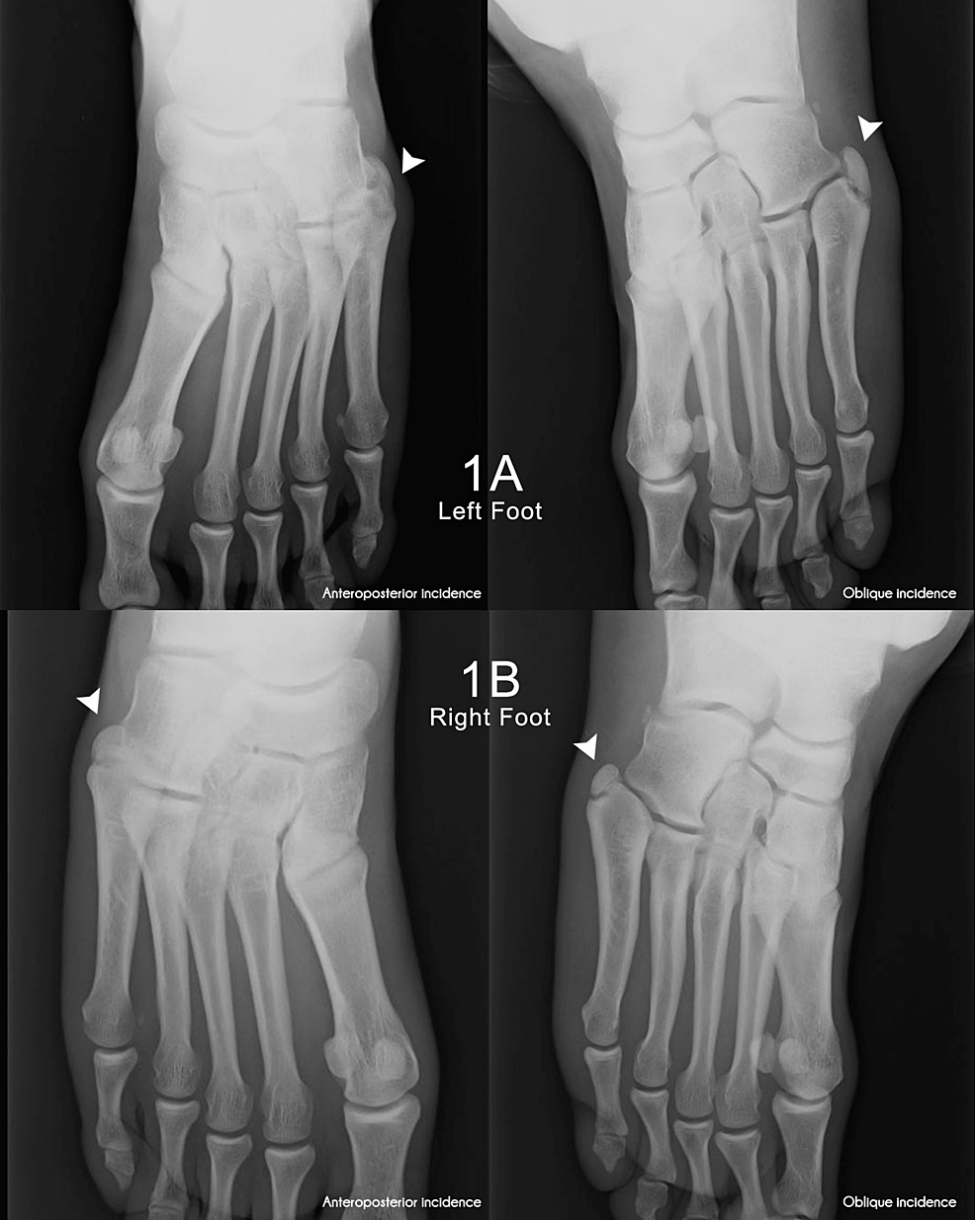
Left (1A) and right (1B) foot anteroposterior and oblique radiographs 1A Left: Anteroposterior incidence of the left foot, 1A Right: Oblique incidence of the left foot, 1B Left: Anteroposterior incidence of the right foot, 1B Right: Oblique incidence of the right foot Os vesalianum pedis is seen on all radiographs (white arrow)
